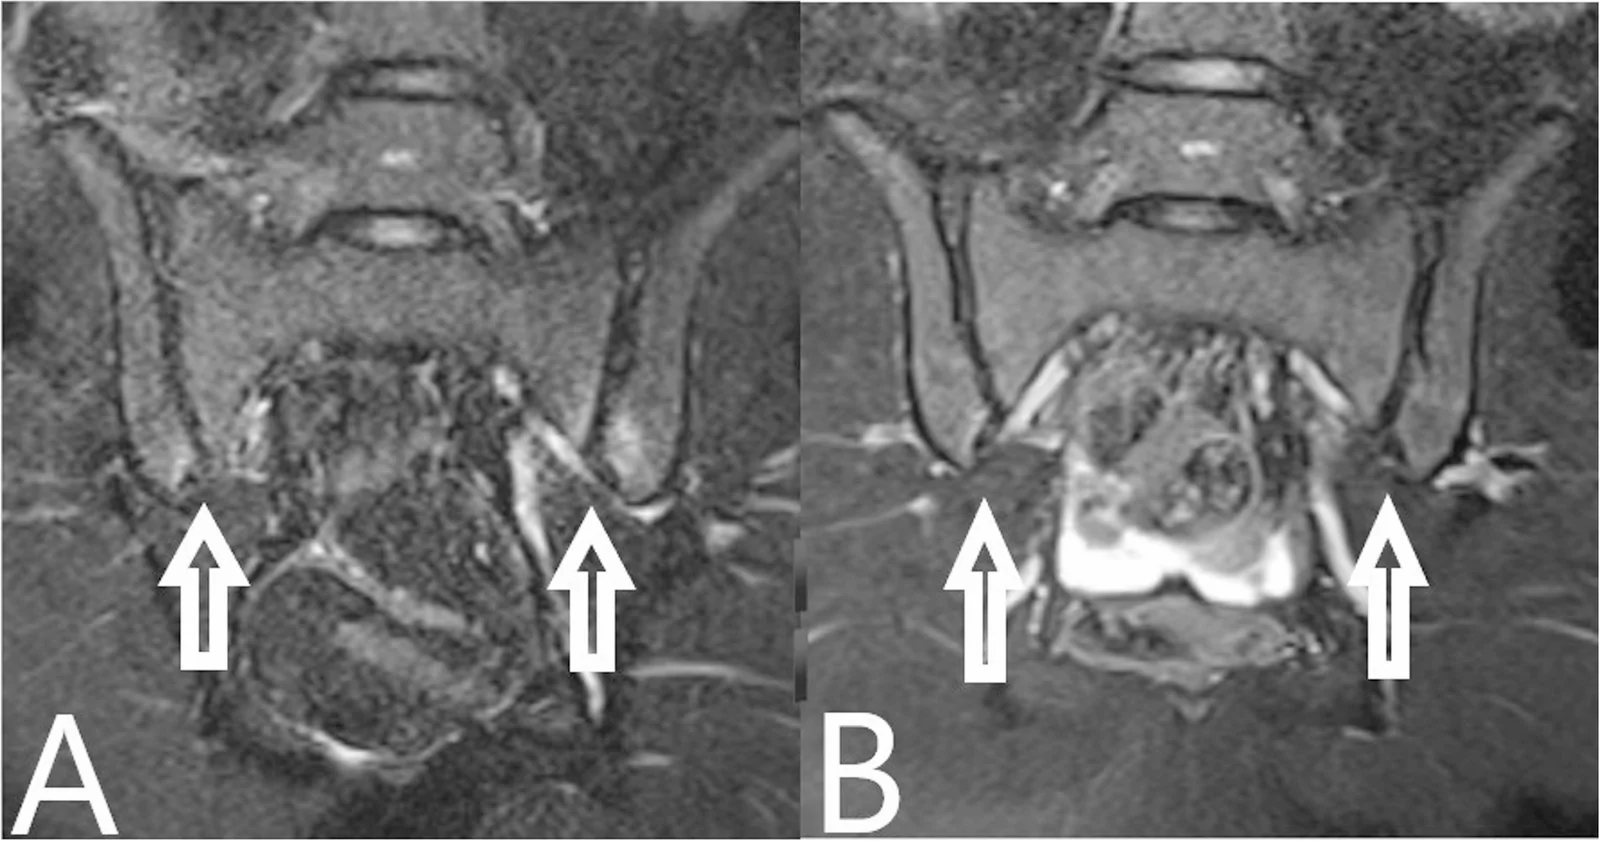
Magnetic resonance imaging (MRI) findings of a patient with isotretinoin-induced sacroiliitis. Bone marrow edema (arrow) observed in Image A has completely resolved in the follow-up scan obtained 3 months later (Image B)
